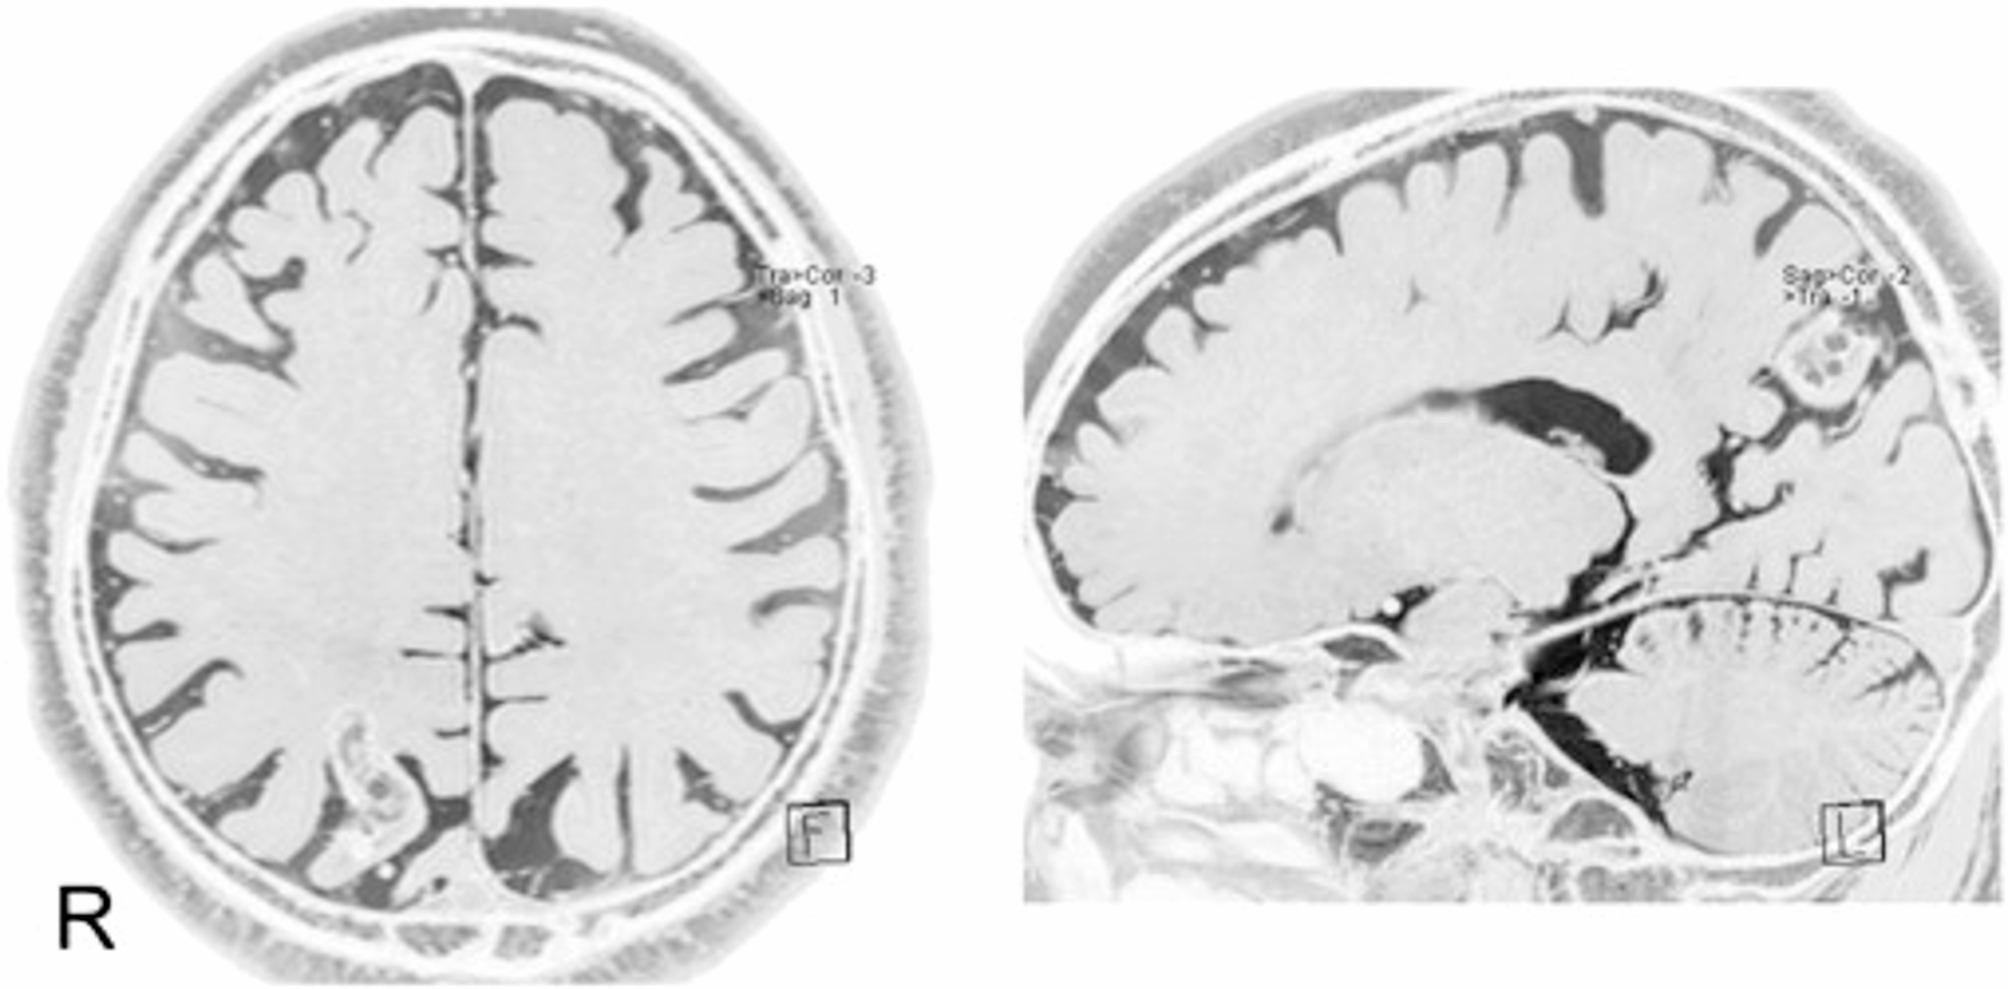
Magnetic resonance imaging. The lesion is visible in the right parietal lobe. Image from Sakurai et al., 2013 [14]. Akinetopsia as epileptic seizure. Epilepsy & Behavior Case Reports, Volume 1, Pages 74–76. © 2013 The Authors. Published by Elsevier Inc. Available under a Creative Commons Attribution 4.0 International License. (CC BY 4.0). No changes were made
